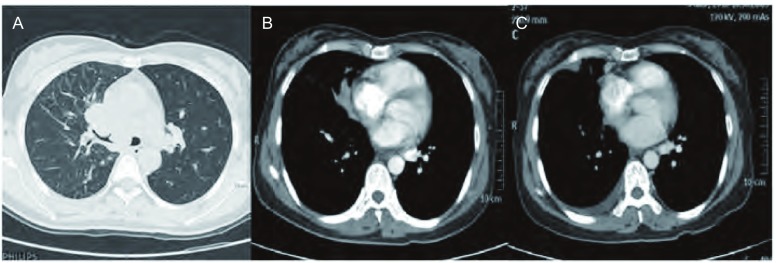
患者女，43岁，肺腺癌伴干性胸膜转移进展为湿性胸膜转移。A：原发灶位于右肺上叶，伴同侧斜裂多发结节状转移；B：胸膜腔内未见积液；C：6个月后，右侧胸腔内示弧形液性密度影，胸水中查到腺癌细胞。 Femal, 43-year-old, follow-up CT images show DPD with eventual pleural effusion in 43-year-old woman with lung adenocarcinoma. A: The primary tumor located in the right upper lobe, with multiple nodal metastases within the ipsilateral major fissure; B: Mediastinal window of CT image (5-mm section thickness) shows no pleural effusion; C: CT image obtained at a similar level and 6 months after A shows a small amount of right pleural effusion, and check lung adenocarcinoma cell by hydrothorax exfoliative cytologic examination.
